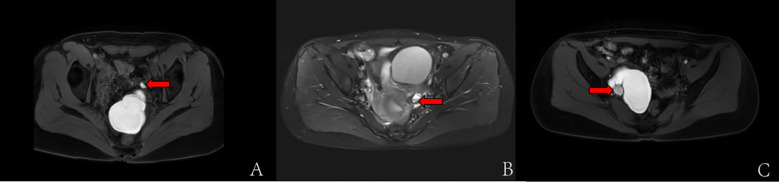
Representative MRI findings in DIE patients. (A) Female, 40 years old, post-operative left ovarian endometrioma, with multiple deep infiltrating lesions visible on the surface of the bowel and omentum (arrow). (B) Female, 38 years old, left ovarian endometrioma, with multiple patchy lesions visible on the posterior uterine wall and peritoneal surface (arrow). (C) Female, 29 years old, bilateral ovarian endometriomas, with multiple pelvic lesions involving the anterior rectal space (arrow). MRI, Magnetic resonance imaging; DIE, Deep infiltrating endometriosis.
